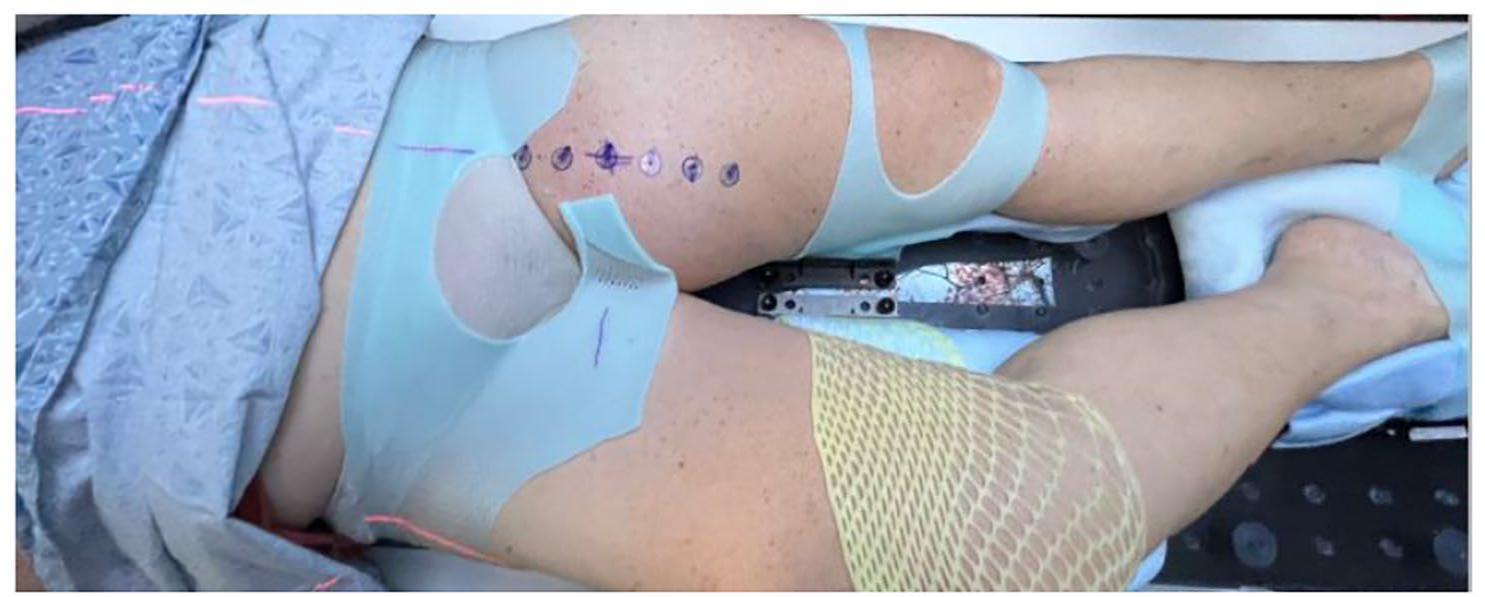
Example of the custom MRI compatible immobilization for a left thigh STS. BB marks on the left anterior thigh will then be tattooed to serve as a guide for setup (e.g., imaging, daily treatment) and habitat directed biopsy that will be used for exploratory genomic studies
